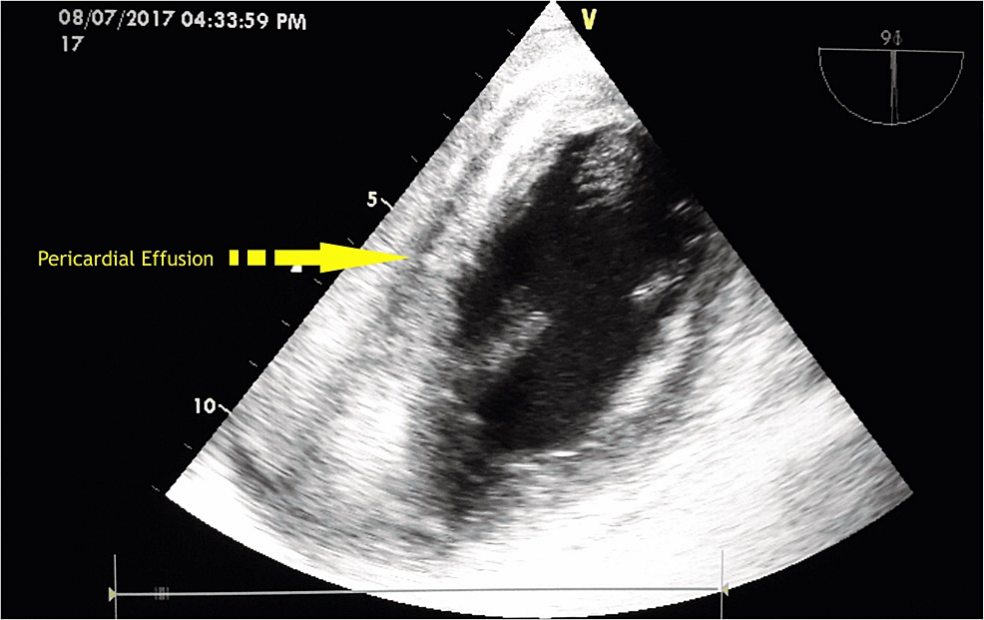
Echocardiographic image showing pericardial effusion
